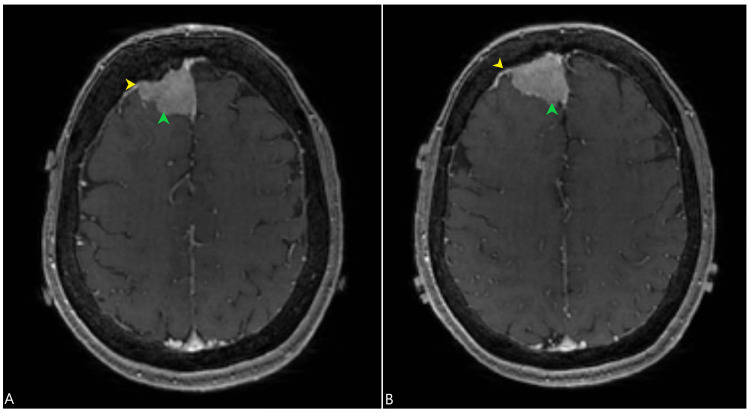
Post-contrast (gadolinium-based) axial MR imaging of the brain showing a right frontal parasagittal meningioma (green arrowheads) along with hyperostosis of the right frontal bone (yellow arrowheads) shown in two different MR sections (A and B). MR: magnetic resonance
